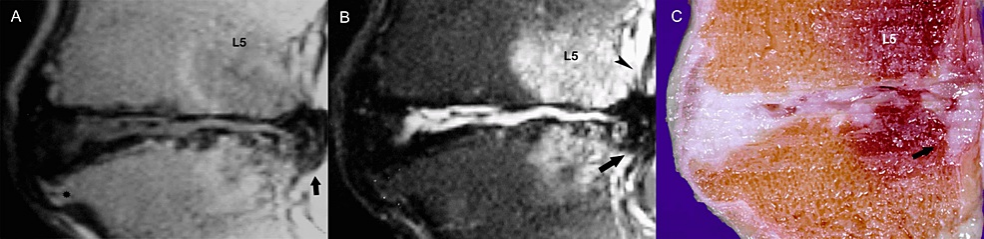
Disc herniation. (A) Sagittal T1-weighted MR image shows severe disc degeneration at the L5-S1 vertebral space with posterior disc herniation (arrow). There is markedly narrowed intervertebral disc space and grossly abnormal configuration of the vertebral end-plates. Note the prominent osteophyte at S1 (asterisk). (B) Sagittal T2-weighted MR image demonstrates extruded disc material (arrow) violating the posterior longitudinal ligament (arrowhead). (C) The findings of intervertebral (osteo)chondrosis are confirmed on the corresponding anatomic section (arrow).
